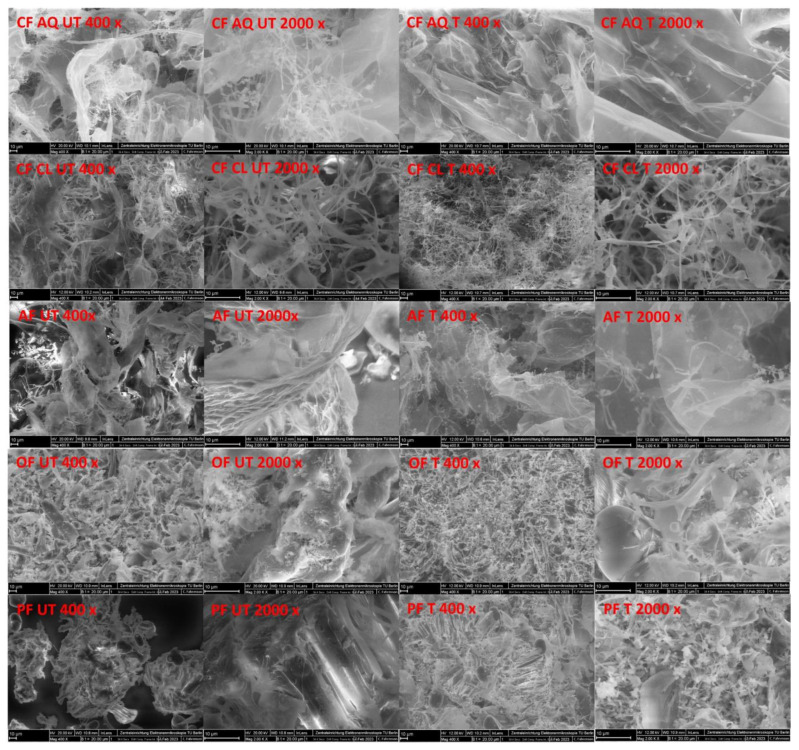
FE-SEM images (400× and 2000× magnification, scale bar 10 µm) of untreated (UT) and ultrasound-treated (T) fiber samples. (CF AQ—Citrus fiber AQ plus, CF Cl—Citrus fiber classic; AF—Apple fiber; OF—Oat fiber, PF—Pea fiber).
